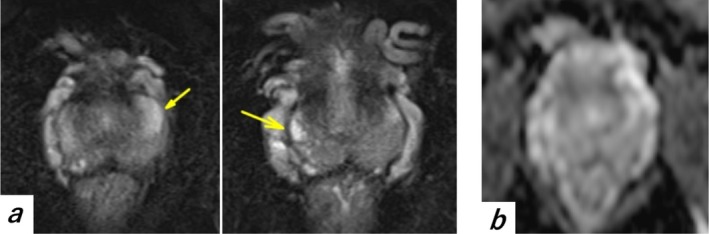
Pelvic magnetic resonance imaging (MRI) of the prostate. (a) T2‐weighted image showing high‐intensity signals (arrows) in the peripheral zone of both lobes, suggestive of inflammation. (b) Apparent diffusion coefficient (ADC) map showing no diffusion restriction in the same region, which is inconsistent with malignancy.
